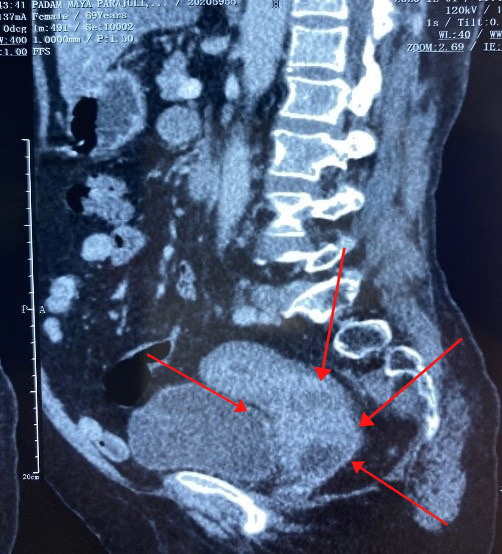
CT scan showing 9x6x5 cm involving the cervix.
